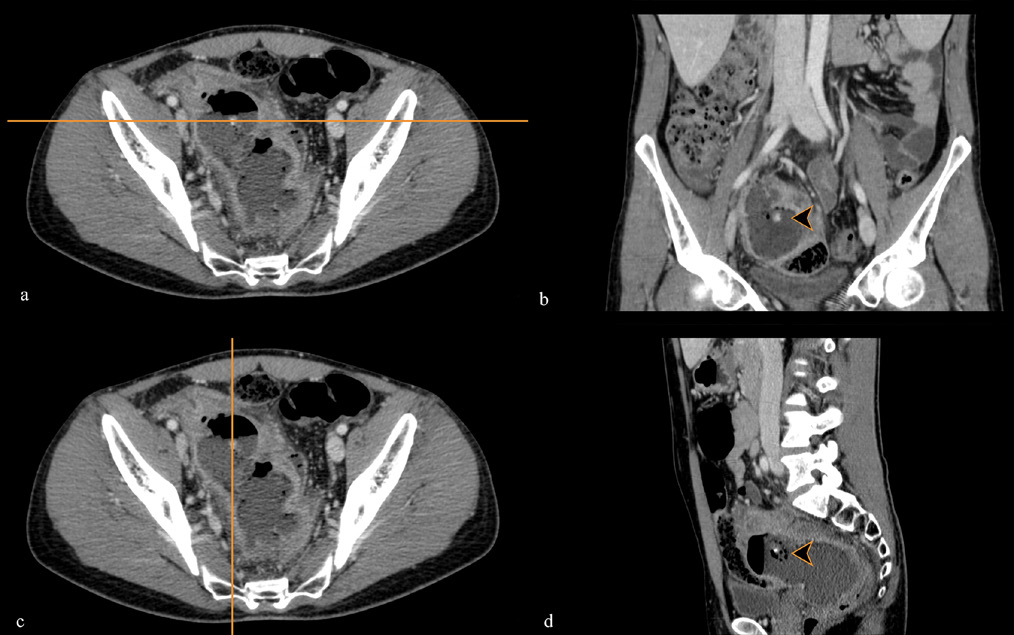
Post-contrast CT scan in portal venous phase: Multiplanar Reconstruction (MPR) images obtained at the level of the subcentimetric appendicolith (arrowheads) inside the abscess along the axial plane (a, c), coronal plane (b) and sagittal plane (d).
